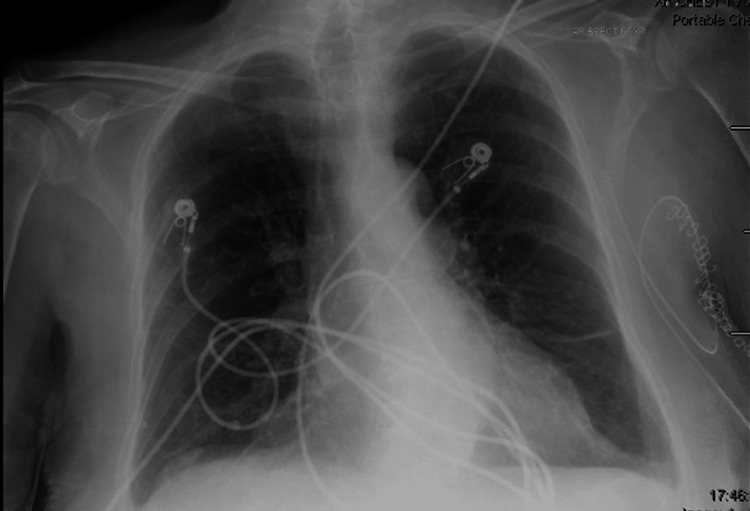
Chest X-ray taken on initial presentation in the Emergency Room. There was no evidence of cardiomegaly, interstitial or lobar infiltrates, cephalization of pulmonary vessels, or other findings consistent with heart failure.
